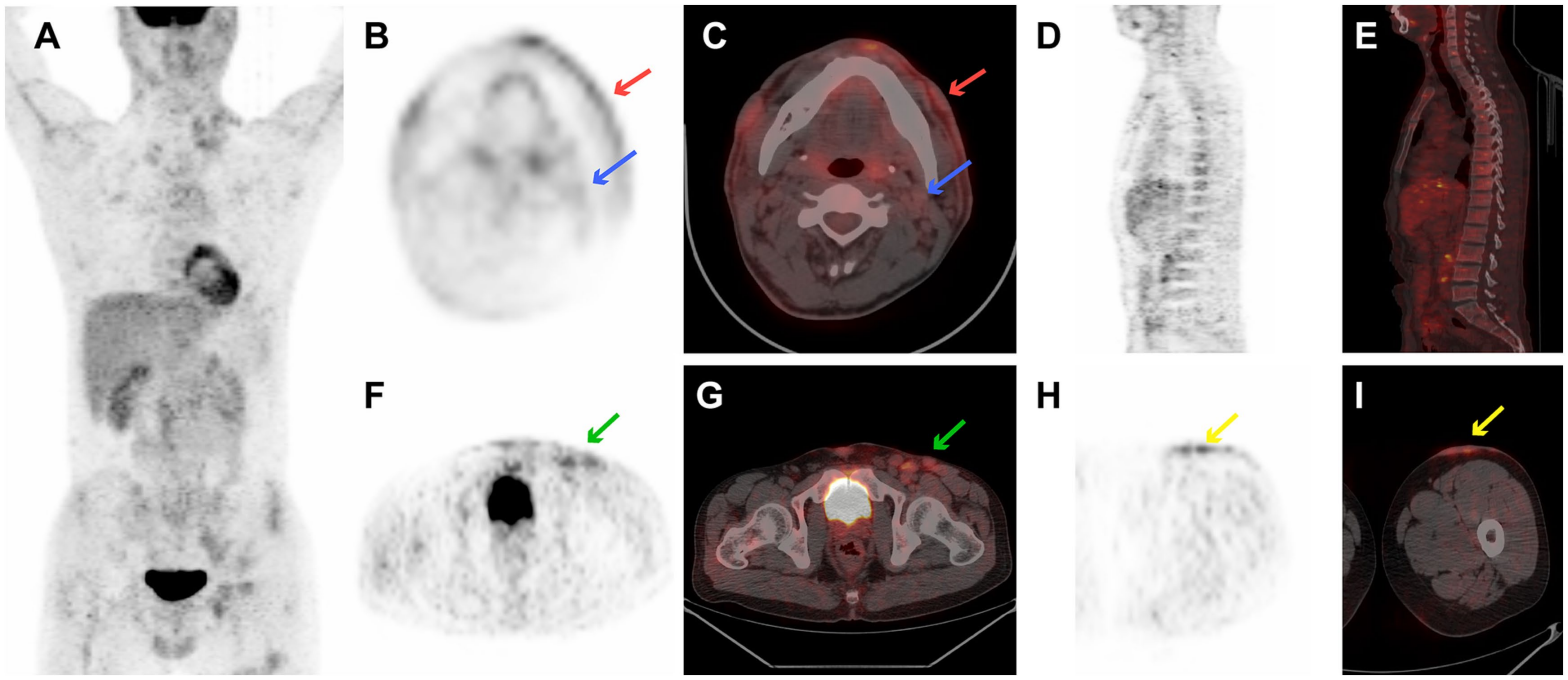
^18^F-FDG PET/CT images of case 4 revealed multiple mild-to-moderate FDG-avid cutaneous lesions on the head, neck, chest, back, and left femoral region with an SUV_max_ of 2.6. There are supra- and infra-diaphragmatic abnormally enlarged lymph nodes with an SUV_max_ of 3.7. Mild FDG-avid of trunk bone was detected with a SUVmax 1.5. (A) PET maximum intensity projection (MIP), (B) transverse PET image of cutaneous lesion of cheek (red arrows) and cervical lymph nodes (blue arrow), (C) transverse fused PET/CT image of cervical cutaneous lesion of cheek (red arrows) and cervical lymph nodes (blue arrow), (D) sagittal PET image of bone, (E) sagittal fused PET/CT image of bone, (F) transverse PET image of left inguinal lymph nodes (green arrow), (G) transverse fused PET/CT image of left inguinal lymph nodes (green arrow), (H) transverse PET image of cutaneous lesion in left femoral region (yellow arrows), and (I) transverse fused PET/CT image of cutaneous lesion in left femoral region (yellow arrows).
